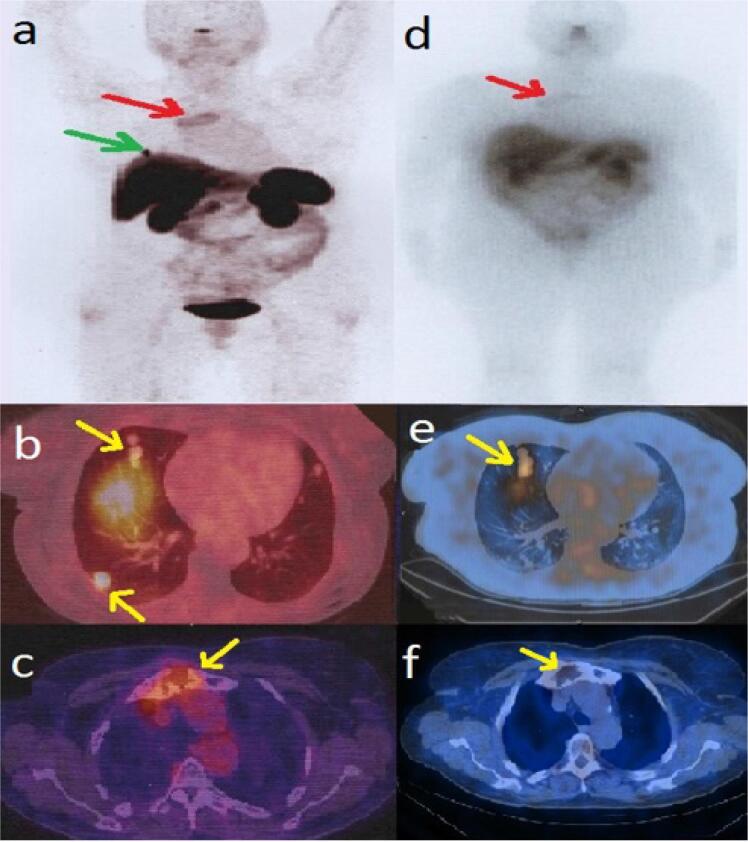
Ga-68-DOTATE MIP ( a ) and PET/CT ( b, c ) confirmed the lesions detected by FDG PET/CT. Therefore, the patient received 200 mci of lu177 DOTA-TATE and performed post-treatment WBS ( d ). lu177 DOTA-TATE SPECT/CT showed increased uptake in pulmonary nodules ( e ) and uptake in the margin of lytic lesion of the sternum (f ).
